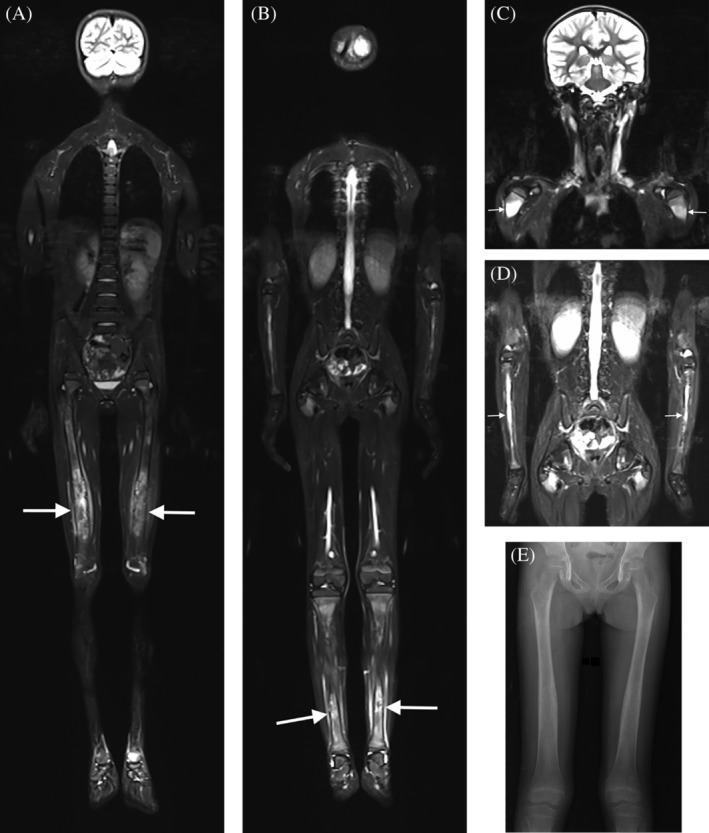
Whole‐body magnetic resonance imaging in Camurati–Engelmann disease. Whole‐body short tau inversion recovery in the coronal plane shows symmetrical edema in the bone marrow of the diaphyses and metadiaphyseal regions of the bilateral femur (arrows in *A*) and tibia (arrows in *B*). Segmental images of the superior parts of the body show edema in the bone marrow of the diaphyses and metadiaphyseal regions of the humerus (arrows in *C*) and radius (arrows in *D*). Cortical concentric thickening and irregularities, with diaphyseal enlargement, were also observed. Note that the epiphyseal regions are spared. There was no restricted diffusion. (*E*) A femoral radiograph shows only slight fusiform cortical thickening of the femoral diaphysis.
